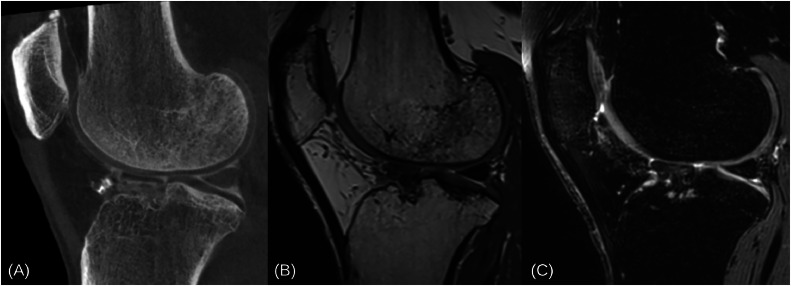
A technically successful CBCT arthrography (A) of the knee joint in a 46-year-old man in sagittal orientation. Intra-articular contrast agent delineates patellofemoral and tibiofemoral cartilage surfaces and reveals the menisci and other intra-articular soft tissue structures (A) similarly to PD-weighted (B) and T2-weighted fat-saturated (C) MRI sequences.
